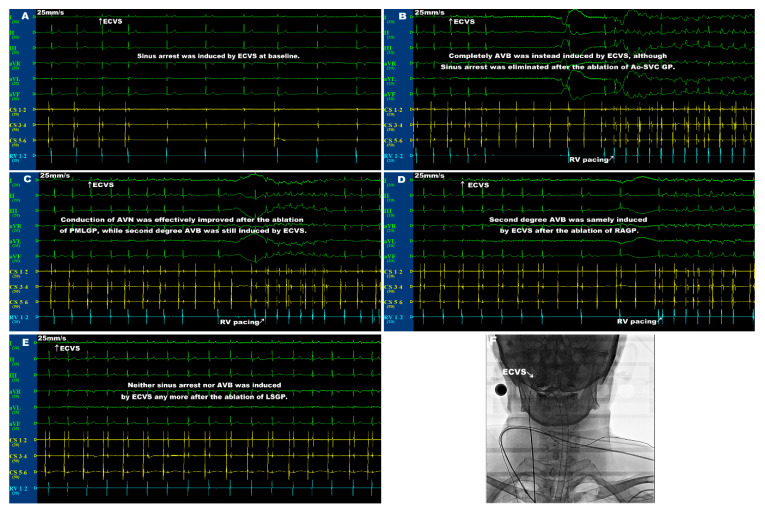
The representative figure of ECVS site and vagal responses of SAN and AVN during the sequential CNA procedure. (A) The vagal response of sinus arrest with a junctional escape rhythm was induced by ECVS at baseline. (B) After the ablation of Ao-SVC GP, the vagal response of sinus arrest evoked by ECVS was effectively eliminated, while complete AVB was instead induced. (C) After the sequential ablation of PMLGP, the previously induced complete AVB was effectively improved, although the patient still suffered from second-degree AVB. (D) After the further sequential ablation of RAGP, the second-degree AVB was samely induced by ECVS. (E) Neither sinus arrest nor AVB could be induced by ECVS any more after the further sequential ablation of LSGP. (F) X-ray fluoroscopy showed that ECVS was performed using a decapolar steerable electrode catheter within the right internal jugular vein at the level of the right upper wisdom tooth. ECVS = extracardiac vagal stimulation, SAN = sinoatrial node, AVN = atrioventricular node, CNA = cardioneuroablation, AVB = atrioventricular block, GP = ganglionated plexus.
